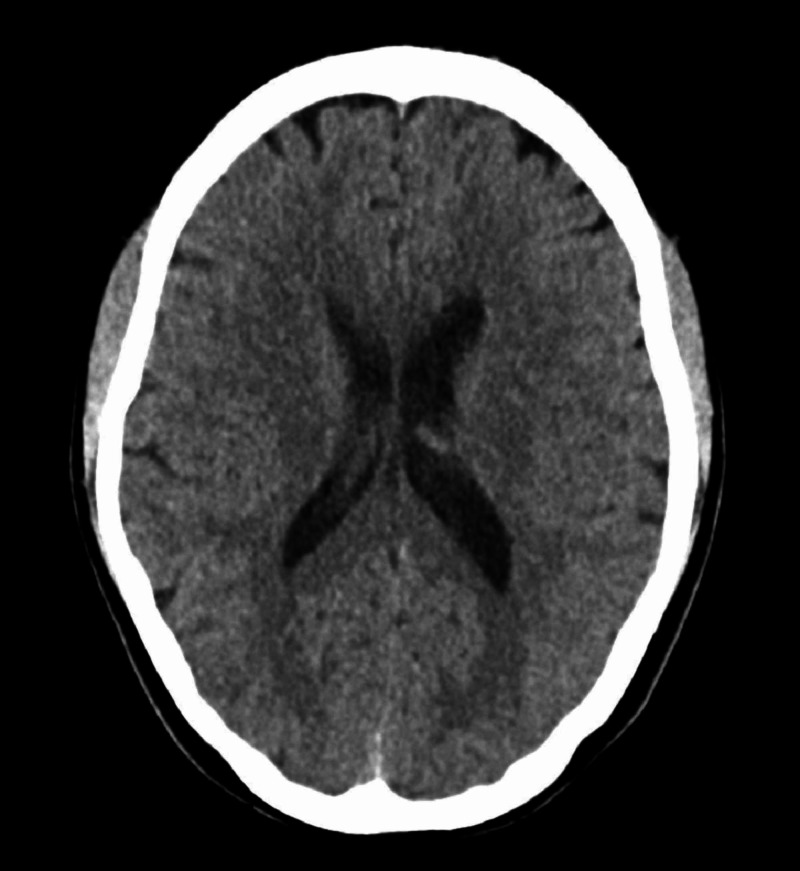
CT head w/o contrast demonstrating no acute findings
